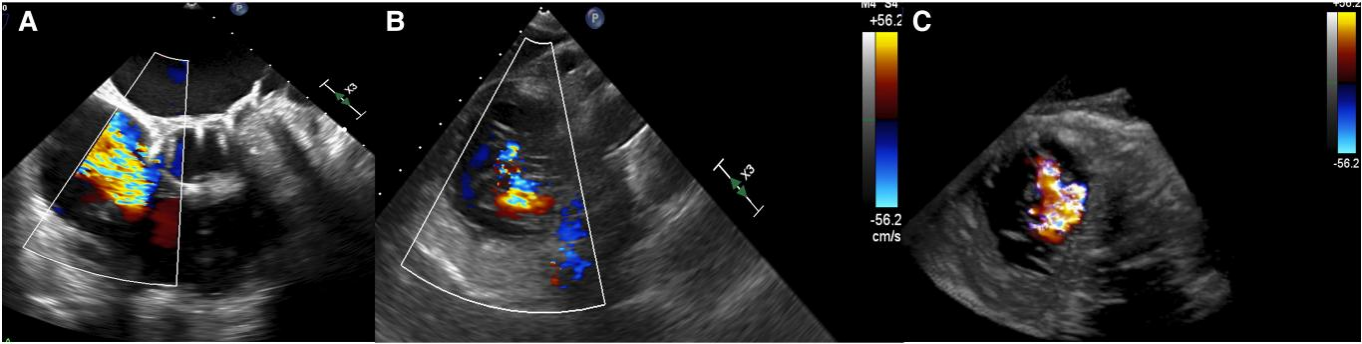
Transoesophageal echocardiography after 1.5 years showing severe tricuspid regurgitation. (*A*) Right ventricle inflow-outflow view; (*B*) transgastric view; (*C*) 3D view. Before TTVI, basal right ventricular diameter was 53 mm with a longitudinal diameter of 76 mm. RV function had a TAPSE of 14 mm with a RV-S′ of 11 cm/s and a RV-FAC of 37.5%.
